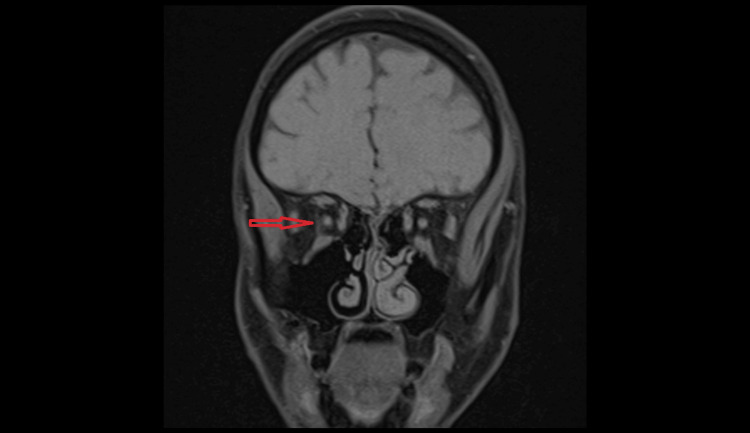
Coronal view of a T1-weighted MRI showing an abnormal, elongated, round structure (red arrow) running between the superior and inferior rectus muscle just lateral to the right optic nerve with a clear fat plane seen between both structures
